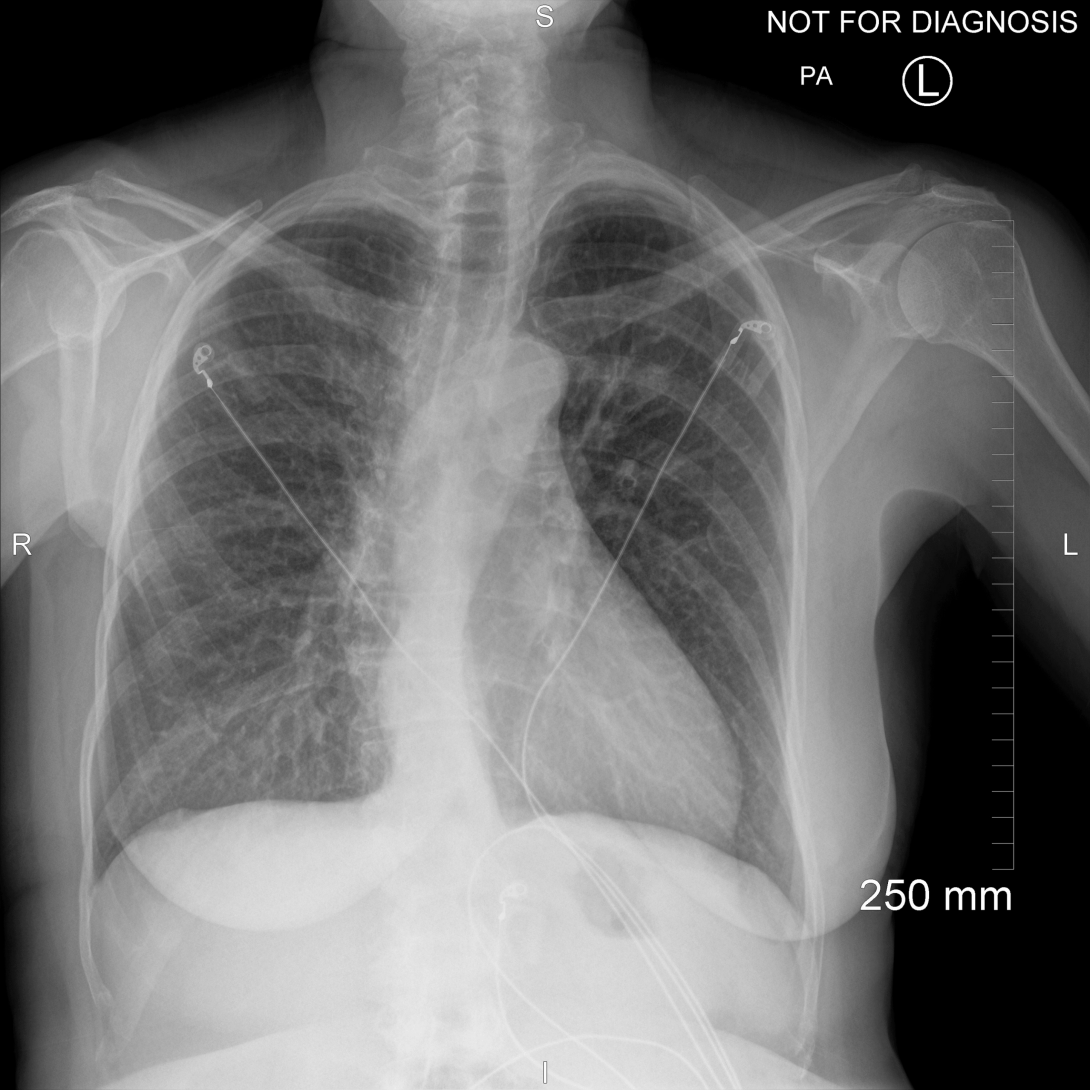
Chest radiograph demonstrated no acute abnormality
